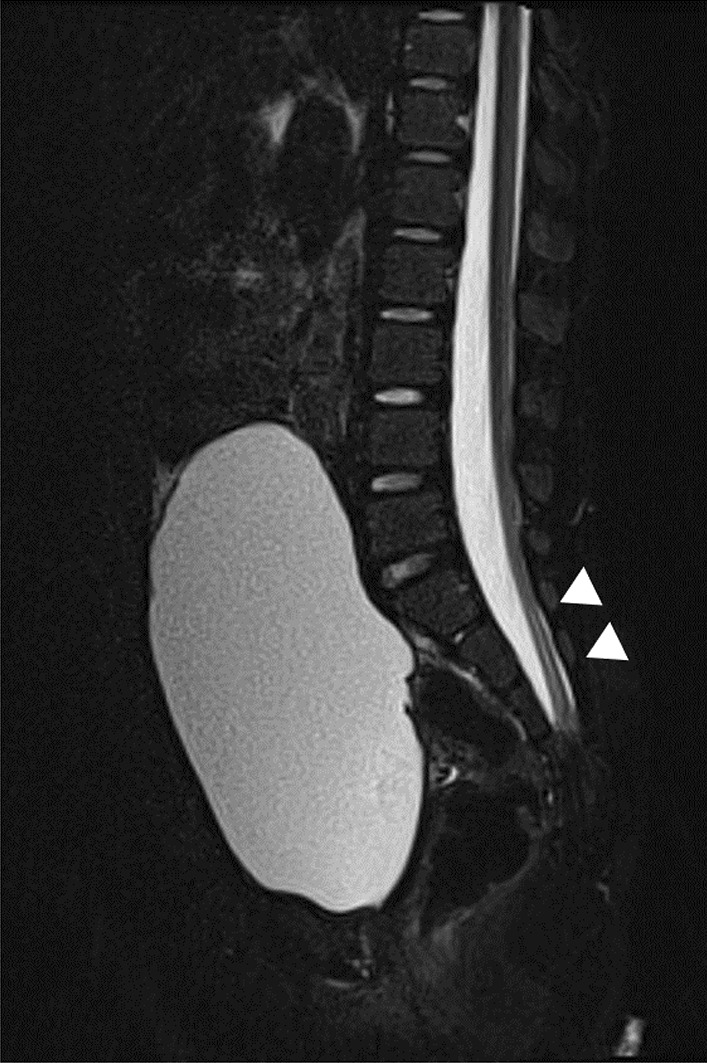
Magnetic resonance imaging of the synsacrum during the first visit. Sagittal T2-weighted image showing tethered spinal cord syndrome and syringomyelia (arrowheads)
